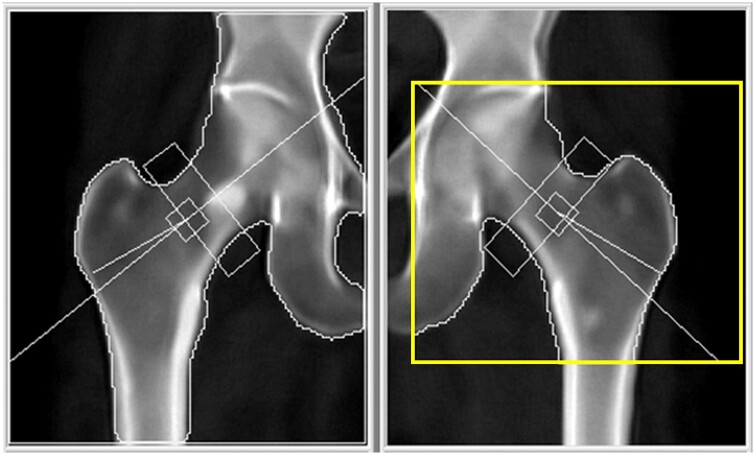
The initial DXA scan of 6-month follow up, the T-scores of the right and left femoral neck were recorded as -0.7 and -2.7, respectively; while d_0_ of the right hip was 56.1, left hip was 54.6. DXA = dual-energy X-ray absorptiometry.
